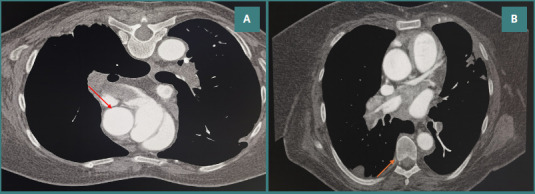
Chest computed tomography pulmonary angiography. A, Axial CTPA image showing a large intraluminal filling defect (red arrow) within the right pulmonary artery, consistent with acute pulmonary embolism. B, Axial CTPA image demonstrating a segmental pulmonary embolism (orange arrow) in the left lower lobe pulmonary artery branch.
